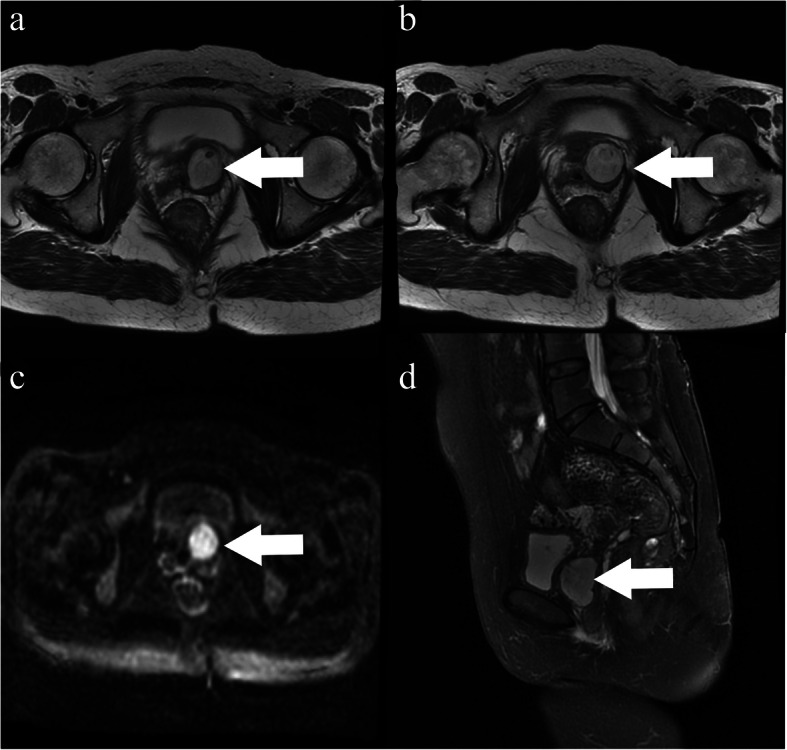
MRI findings of vaginal paraganglioma. a and b: T2WI showed high signal on the left side of the vagina (white arrow); c: the lesion showed high signal on DWI (white arrow); d: T2WI fat suppression phase (sagittal position) showed that the tumor (white arrow) was located behind the bladder, and closely related to the rectum
